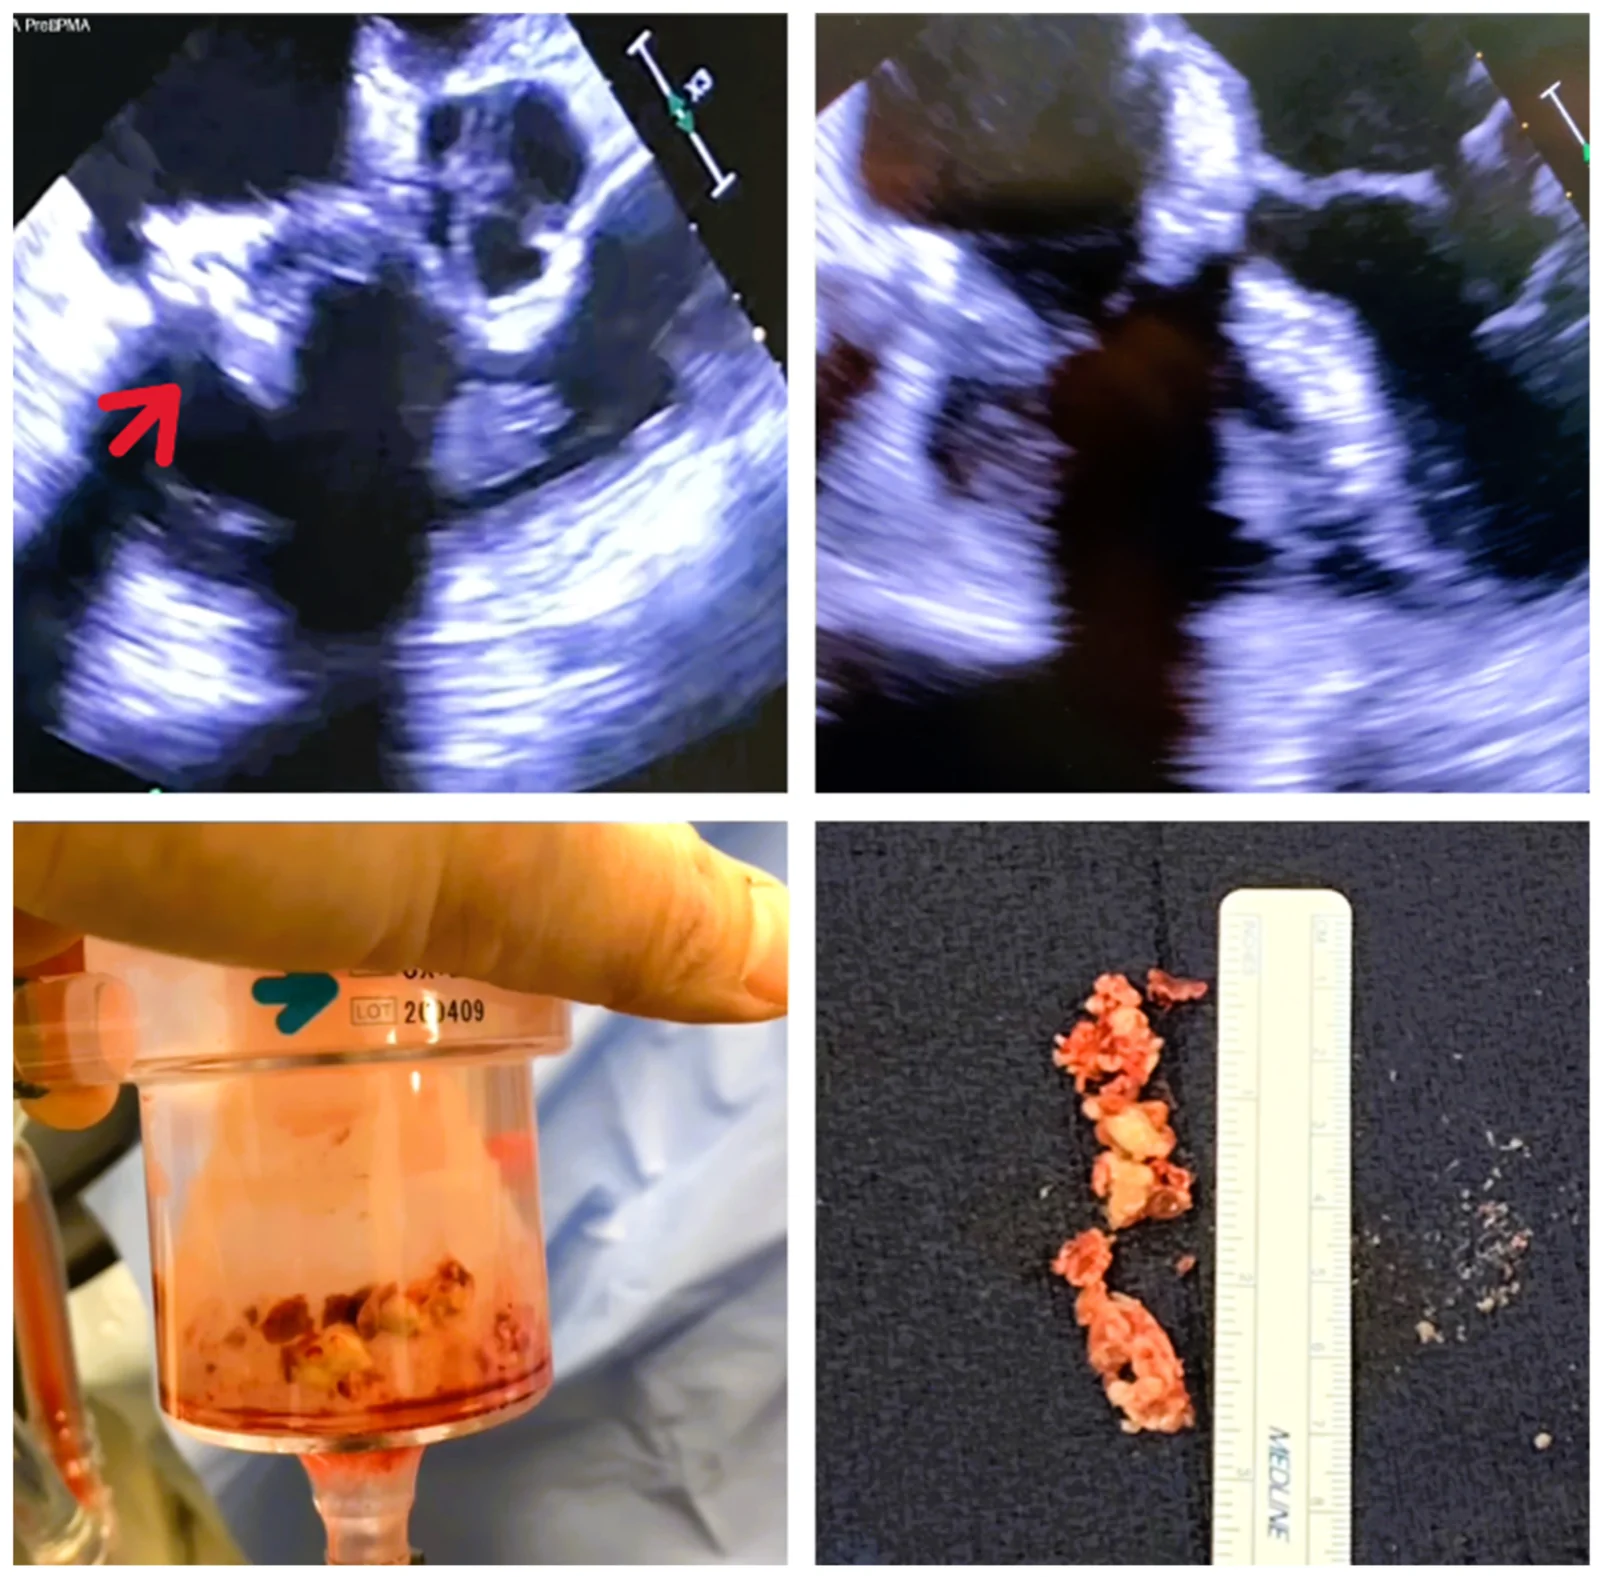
Representative example of vegetation debulking using PMA. Transesophageal echocardiographic mid-esophageal four-chamber view demonstrating a large tricuspid valve vegetation prior to PMA (arrow, (top left)) and post-procedural echocardiographic image demonstrating reduction in vegetation burden following aspiration (top right). Aspirated material is shown in the filter trap (bottom left) and after being flushed out of the trap (bottom right).
